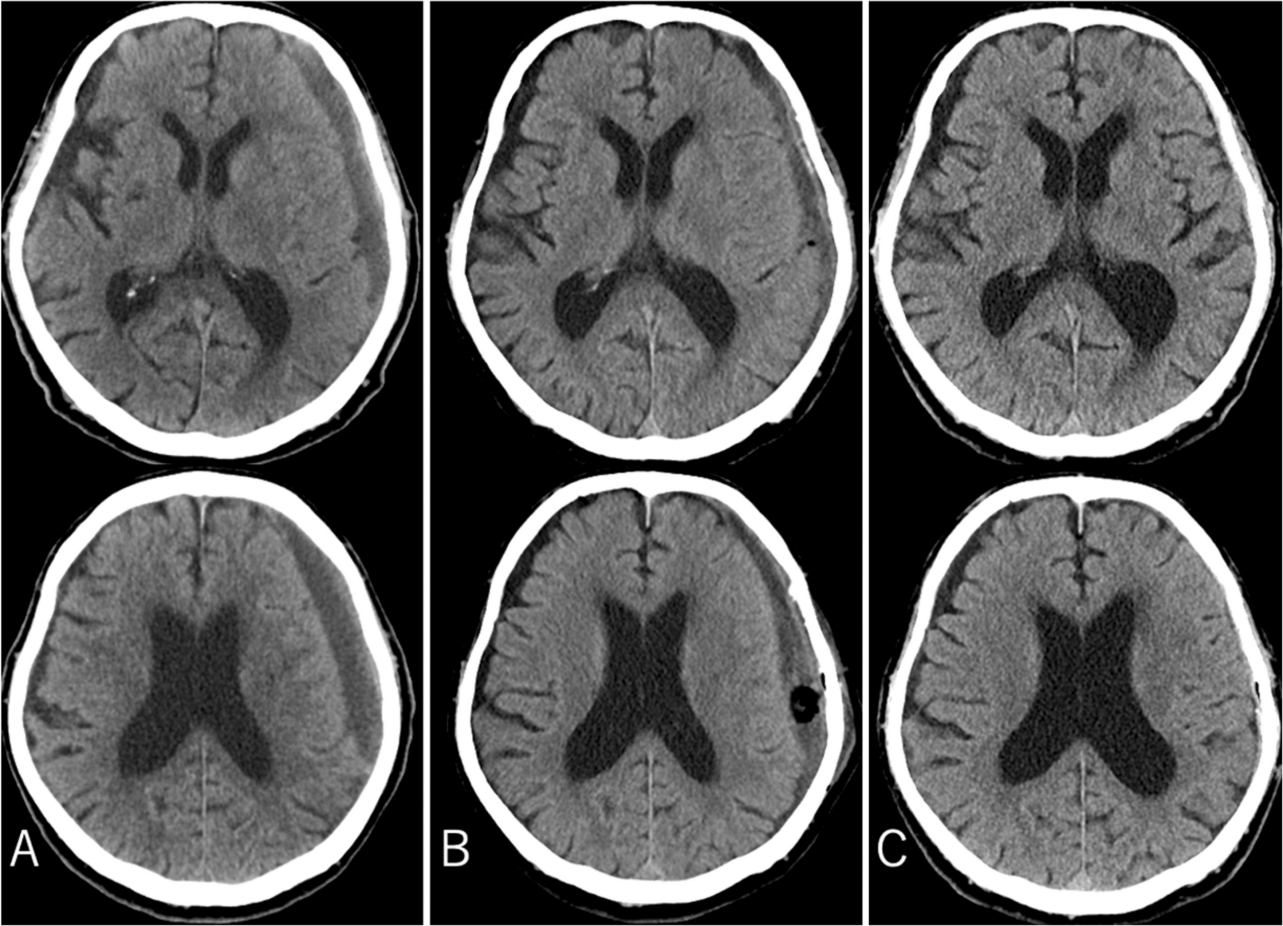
Axial non-enhanced cranial CT images. (A) Two months post-surgery, when the patient developed aphasia, cranial CT revealed a recurrence of the left CSDH. (B) After MMA embolization and a second burr-hole surgery, cranial CT showed that the hematoma had been removed and the cerebral compression had disappeared. (C) The patient has remained asymptomatic for over six months, and a CT scan at six months postoperatively shows no recurrence of CSDH. CT, computed tomography; CSDH, chronic subdural hematoma; MMA, middle meningeal artery
